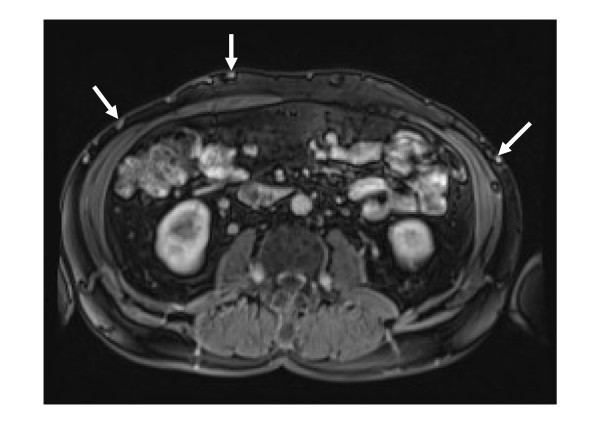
Gadolinium-enhanced MRI showing multiple paraumbilical and abdominal wall veins.
